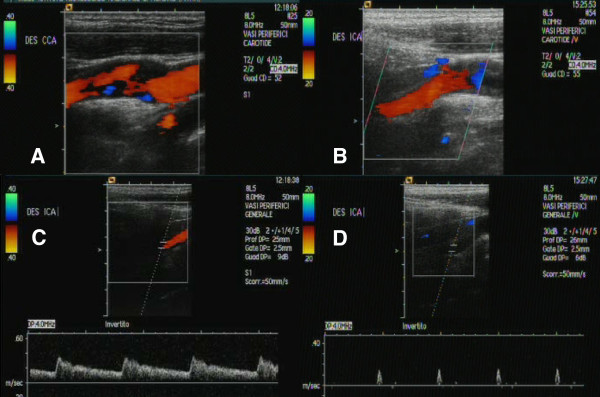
Duplex sonography findings. A. B-mode image performed on 9th March: complete filling of the right ICA lumen with normal PRF. B. B-mode image performed on 14th March: complete filling of the right ICA lumen with normal PRF. C. Doppler flow measurement performed on 9th March: normal waveform. D. Doppler flow measurement performed on 14th March: a waveform with a very low amplitude, high-resistance and no diastolic flow.
